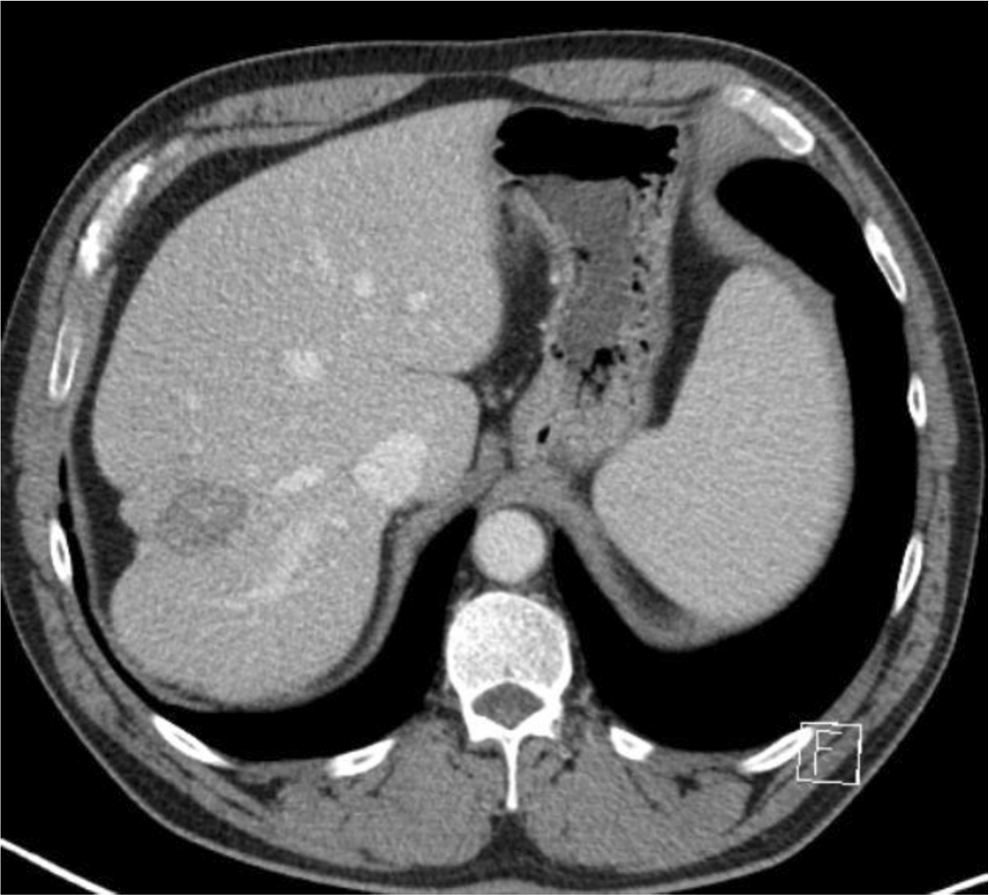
Axial portal venous CT demonstrating capsular retraction in segment 7/8 with a hypo-enhancing ablation zone deep to it
